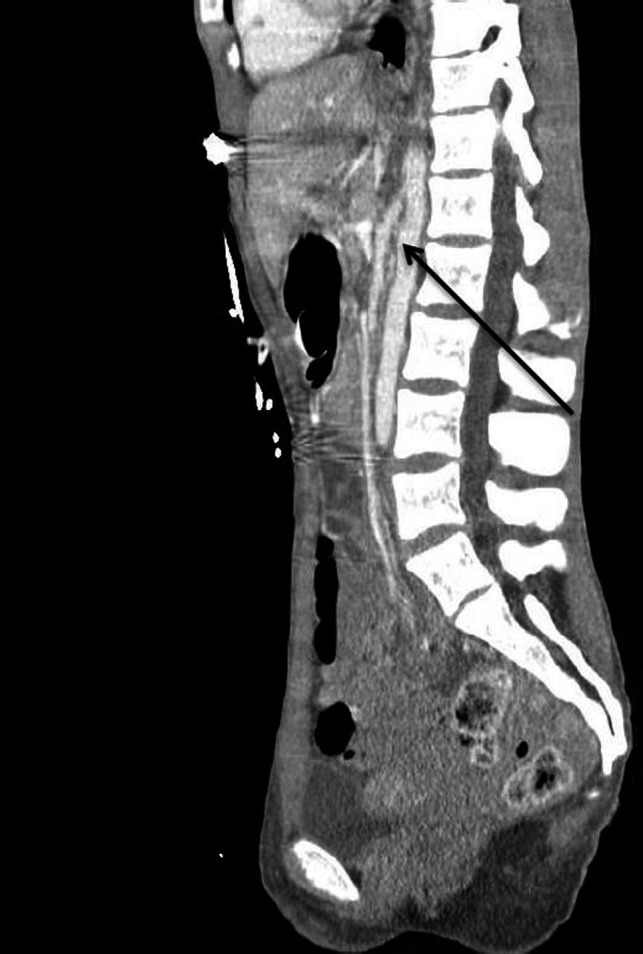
A sagittal image of a computed topography (CT) of the abdomen and pelvis with intravenous contrast showing the narrowed angle between the superior mesenteric artery (SMA) and the aorta
